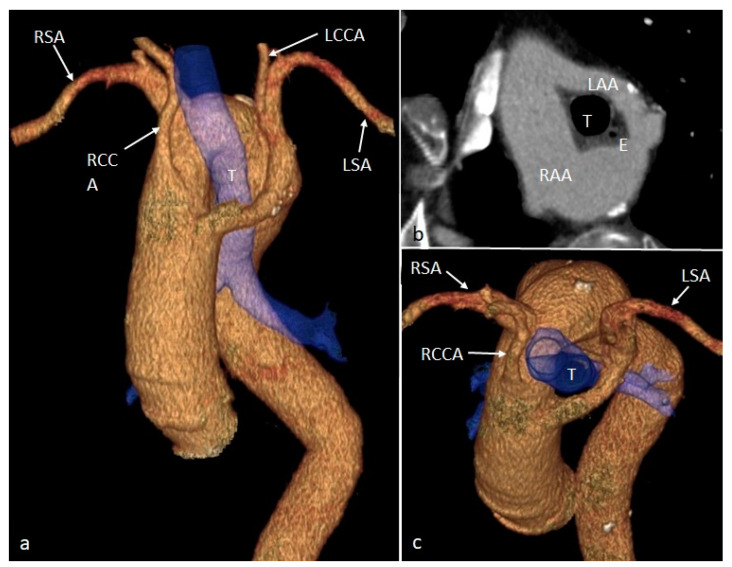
VR (a,c) and oblique CT (b) images showing the double aortic arch (with right dominance—RAA), encircling the trachea (T) and esophagus (E), with a significant stenotic effect on the latter. Each aortic hemiarch (right and left) gives rise to the common carotid and subclavian arteries of the same side. RSA—right subclavian artery. RCCA—right common carotid artery. LCCA—left common carotid artery. LSA—left subclavian artery. RAA—right aortic arch. LAA—left aortic arch. T—trachea. E—esophagus.
